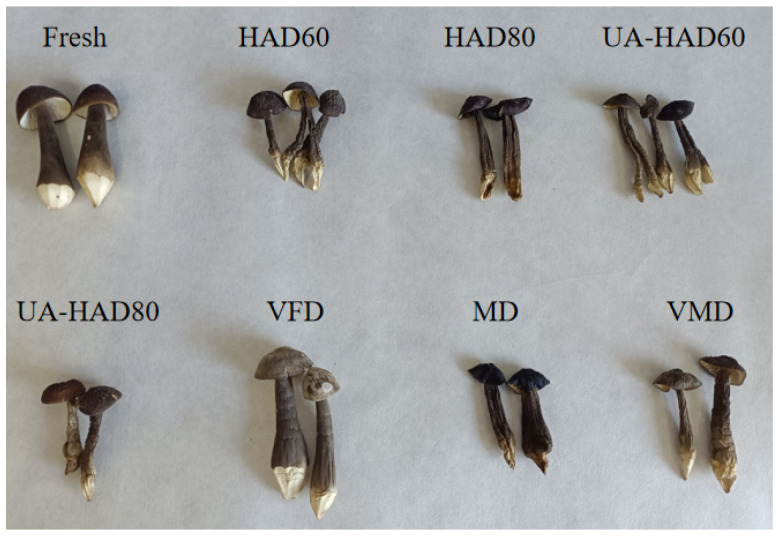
Macroscopic photo of fresh *O. raphanipes* and after drying, HAD60—60 °C hot air drying, HAD80—80 °C hot air drying, UA-HAD60—ultrasonic-assisted 60 °C hot air drying, UA-HAD80—ultrasonic-assisted 80 °C hot air drying, VFD—vacuum freeze drying, MD—microwave drying, VMD—vacuum microwave drying.
